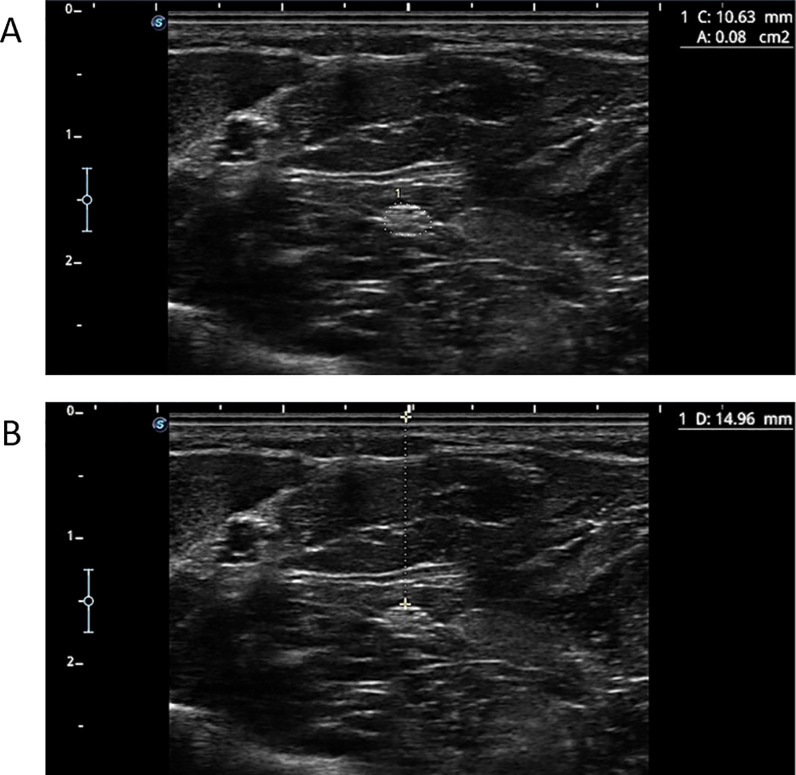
Ultrasound image of the MN where measurements were performed in the middle third of the forearm. The cross-sectional area (CSA) (A) and the nerve-to-skin distance (B) are shown.
